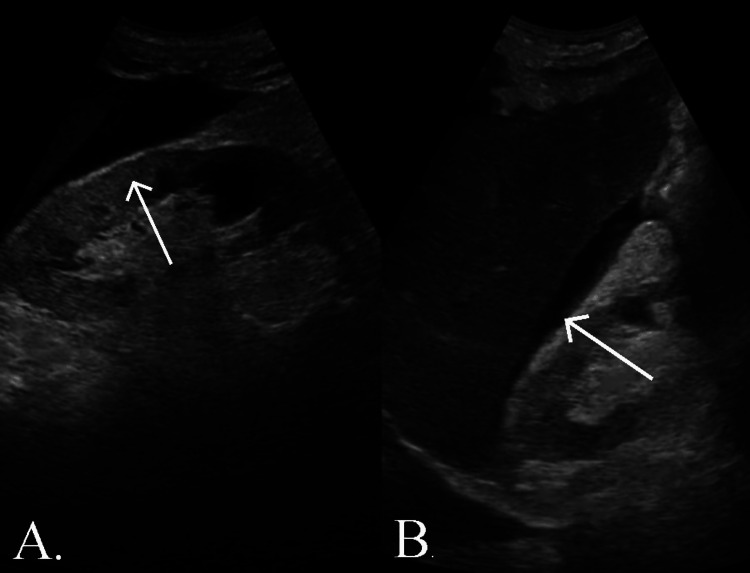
Abdominal ultrasonography demonstrating complications associated with acute fatty liver of pregnancy: (A) free intraperitoneal fluid consistent with ascites (arrow); (B) enlarged liver consistent with hepatomegaly (arrow).
